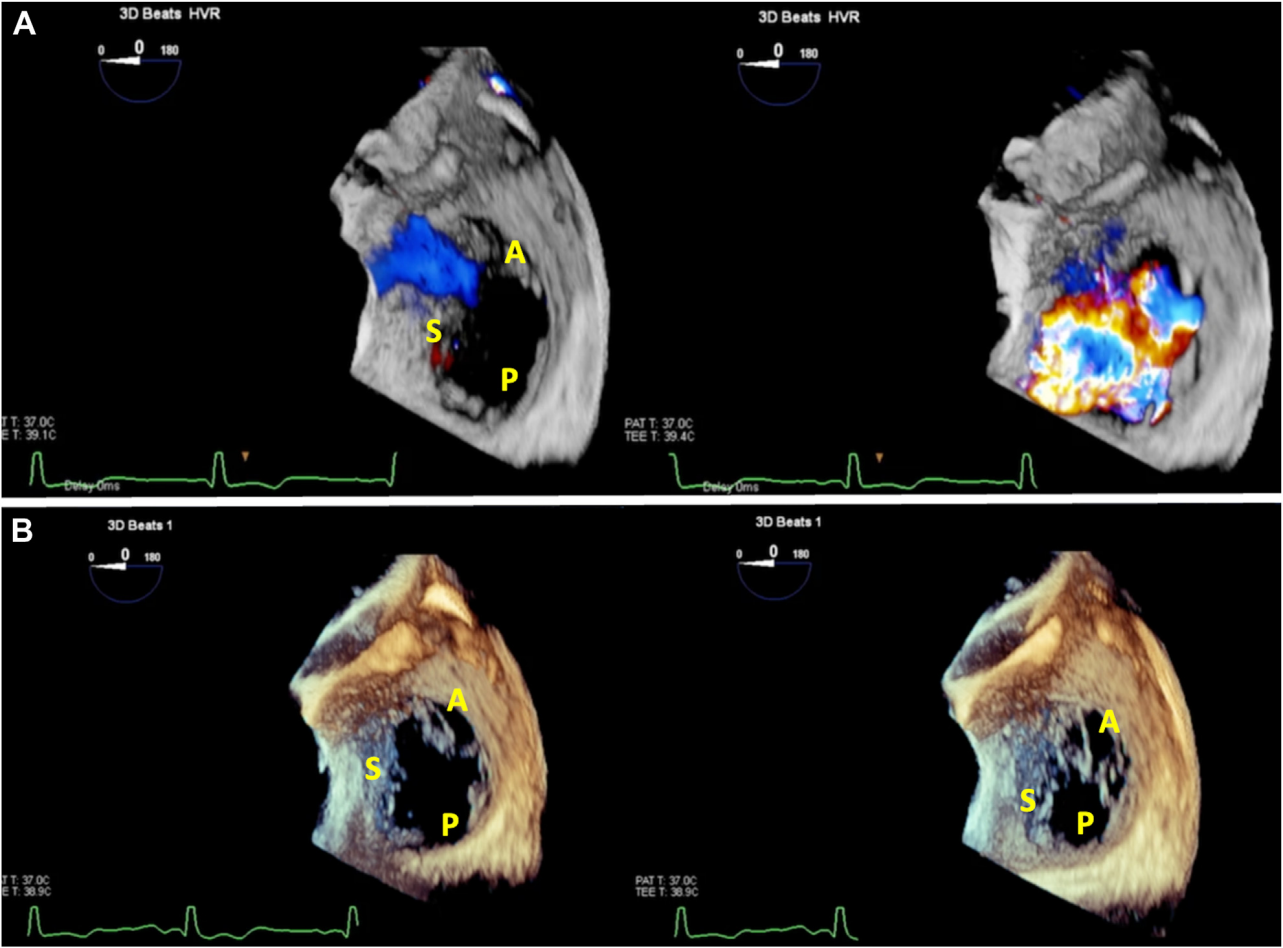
Three-dimensional TEE, single-beat, volume-rendered reconstruction, with (A; *top row*) and without (B; *bottom row*) color-flow Doppler in diastole (*left*) and systole (*right*) demonstrates the severe TR seen passing through the gap created by the hypoplastic posterior leaflet. *A*, Anterior leaflet; *P*, (hypoplastic) posterior leaflet; *S*, septal leaflet.
